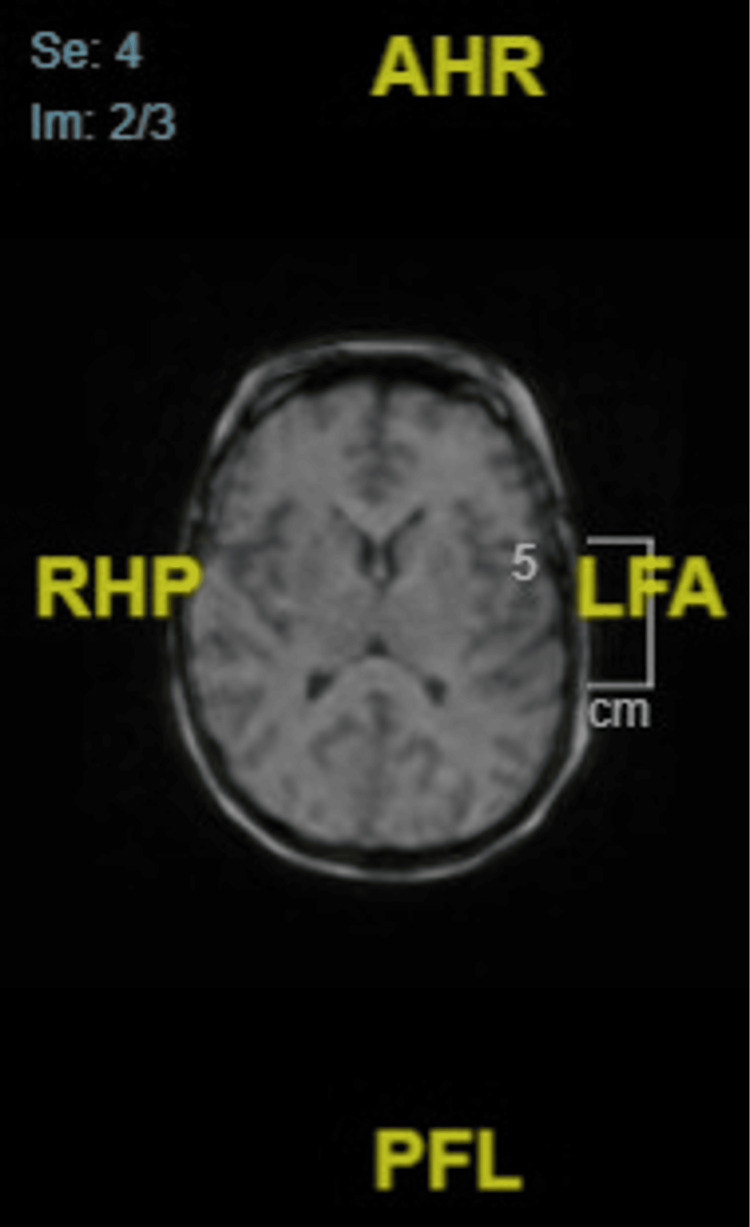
Brain MRI without contrast The patient's brain MRI showed no significant abnormalities or masses MRI: magnetic resonance imaging
